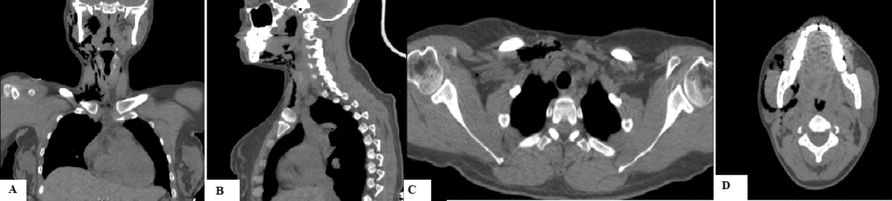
Neck and chest computed tomography shows air accumulation involving: (A), coronal view; in the right side supraclavicular fossa, unilateral neck, and buccal regions; (B), sagittal view; neck and submandibular region; (C), axial view; upper part of chest; (D), axial view, right side buccal region
